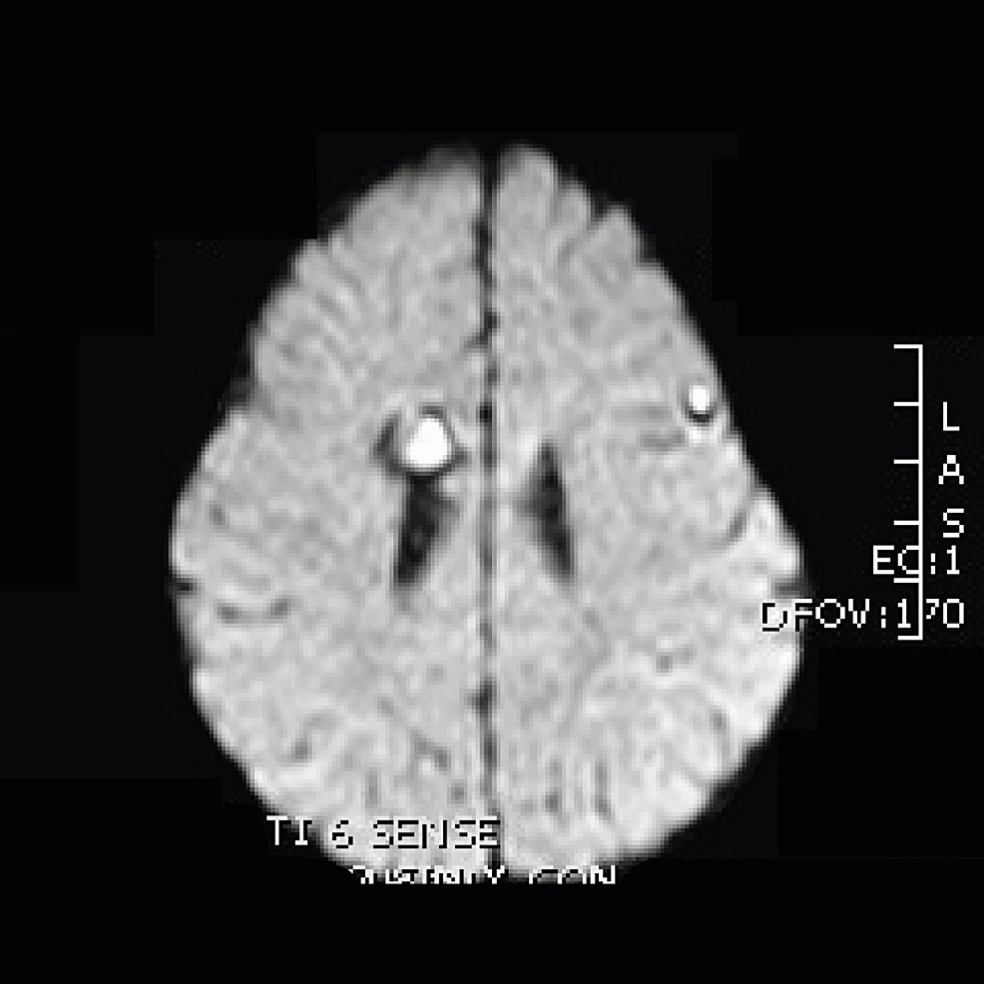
Diffusion-weighted image from head MRI of the five-month-old infant.
